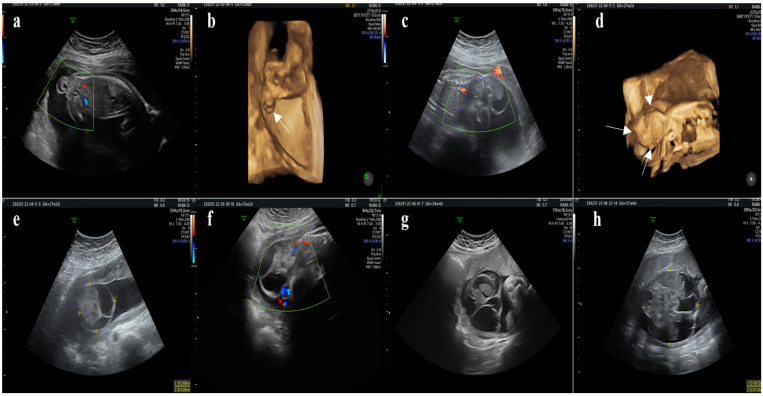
(a–h) 2D/3D ultrasound images at different gestational ages (21, 27, 31, 34, and 37 weeks) show mixed solid and cystic components originating from the coccyx without intrapelvic extension; with no hypervascularity (the white arrows show the teratoma in the 3D reconstruction).
